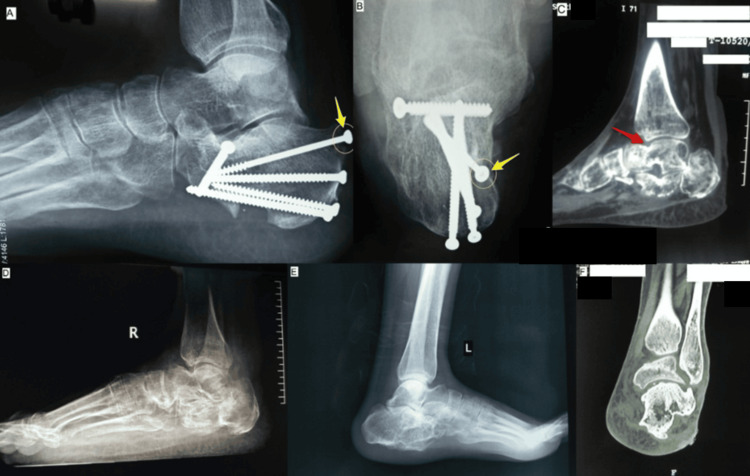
(A, B) X-rays showing the calcaneus fracture treated previously with percutaneous screws - patient complained of pain due to a prominent screw head (yellow circles). (C, D) Dorsiflexed ankle (red arrow) causing anterior tibiotalar impingement. (E, F) Plantar exostoses leading to heel pad pain in the patient
